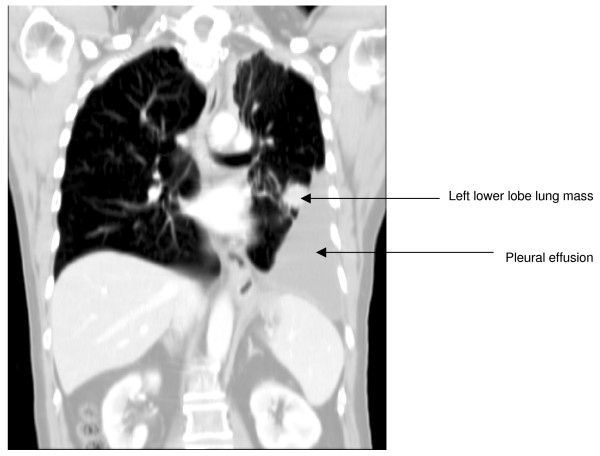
Coronal view of thoracic computed tomography scan showing large left pleural effusion and mass in the left lower lobe.
